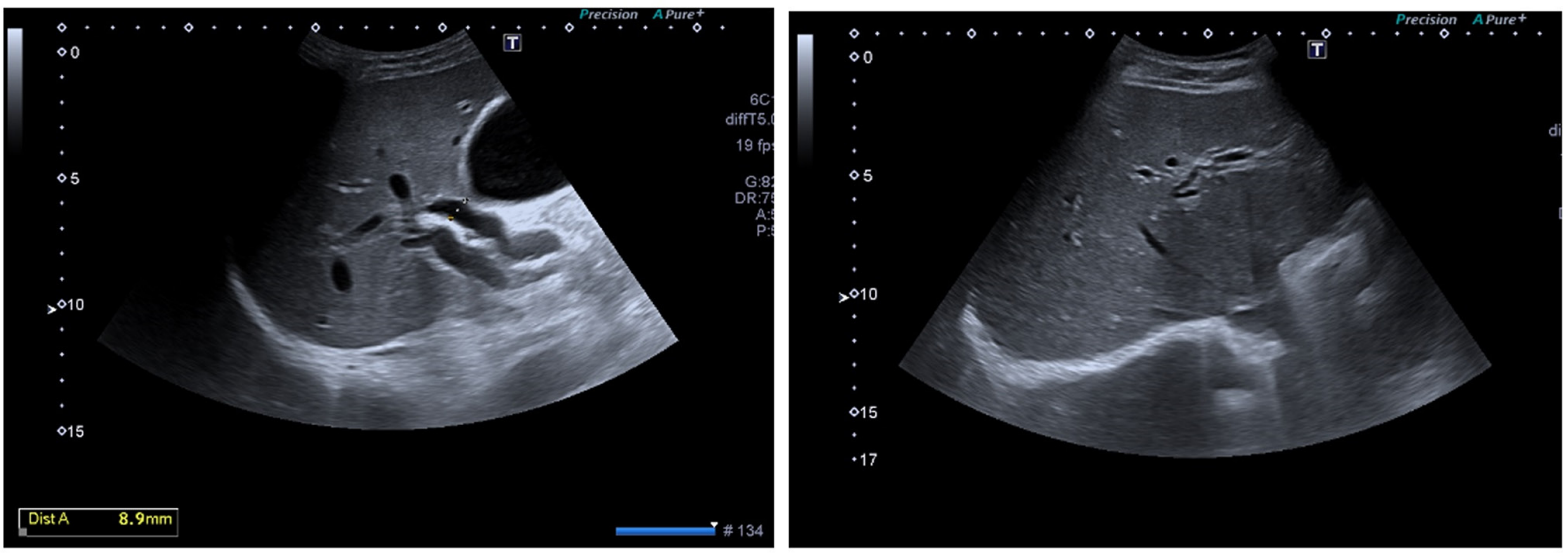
Abdominal ultrasound: acute cholecystitis and intra- and extra-hepatic biliary dilatation.
